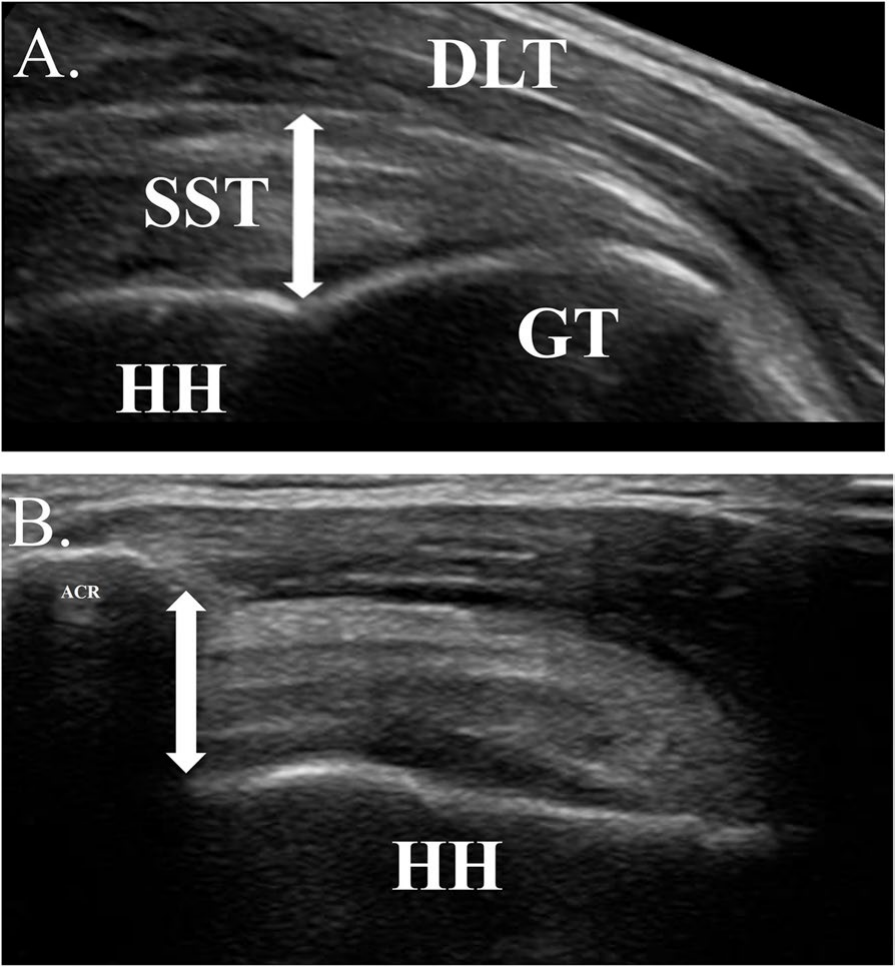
Ultrasound measurement for a) supraspinatus tendon thickness (double-ended arrow), and b) acromiohumeral distance (double-ended arrow). SST: Supraspinatus tendon, DLT: Deltoid muscle, HH: Humeral head, GT: Greater tuberosity, ACR: Acromion
